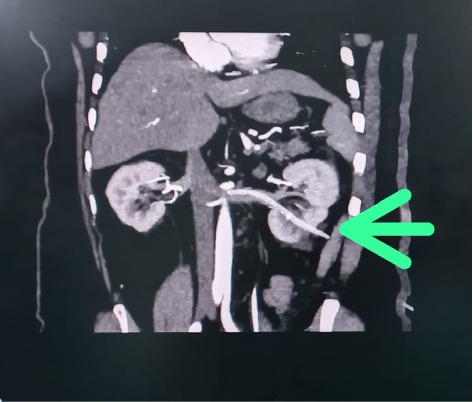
CT indicating nephrostomy tube in left renal vein.
